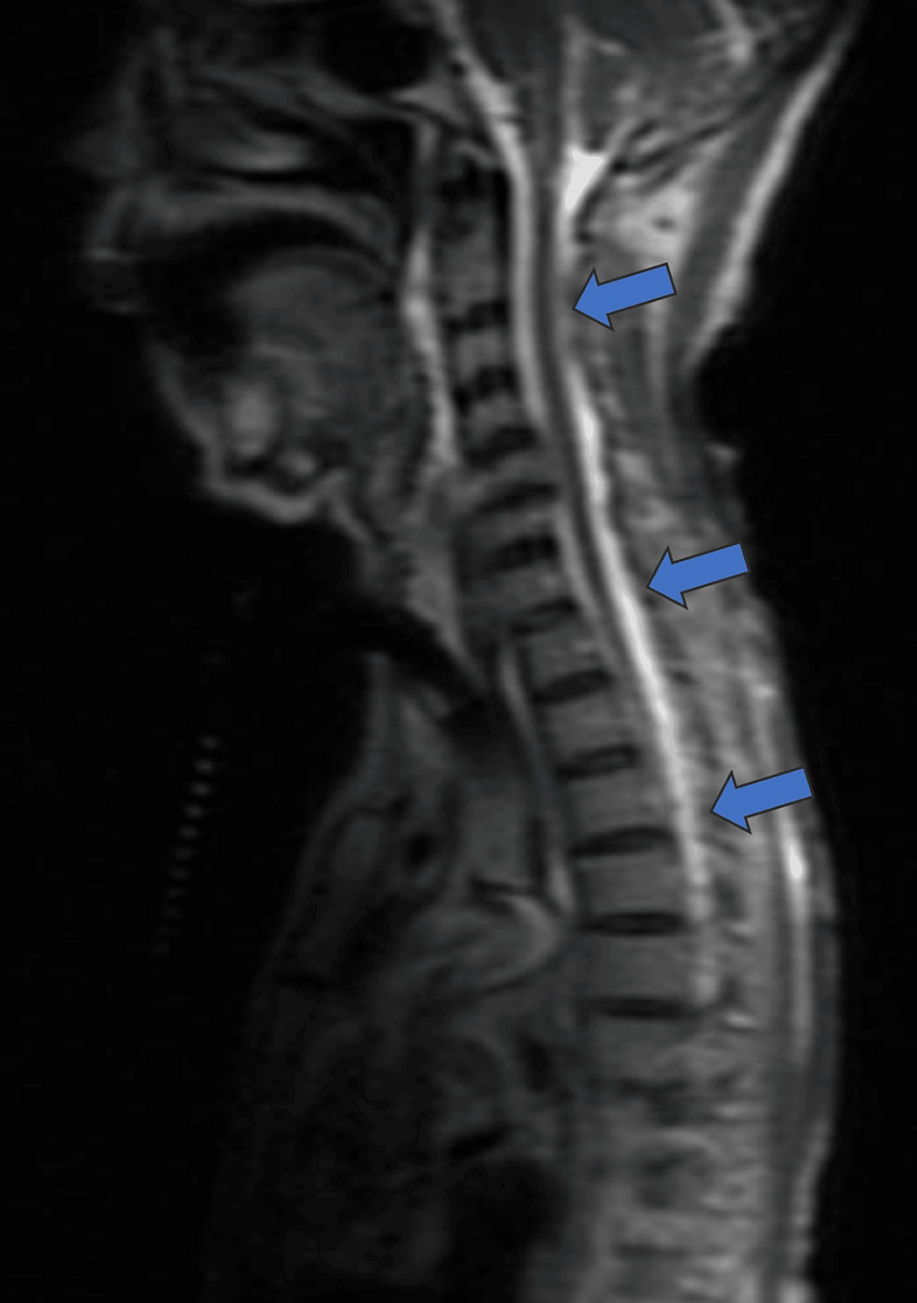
Magnetic resonance imaging (MRI) of spine showing spinal levels C3-T10 subdural collections (arrows)
